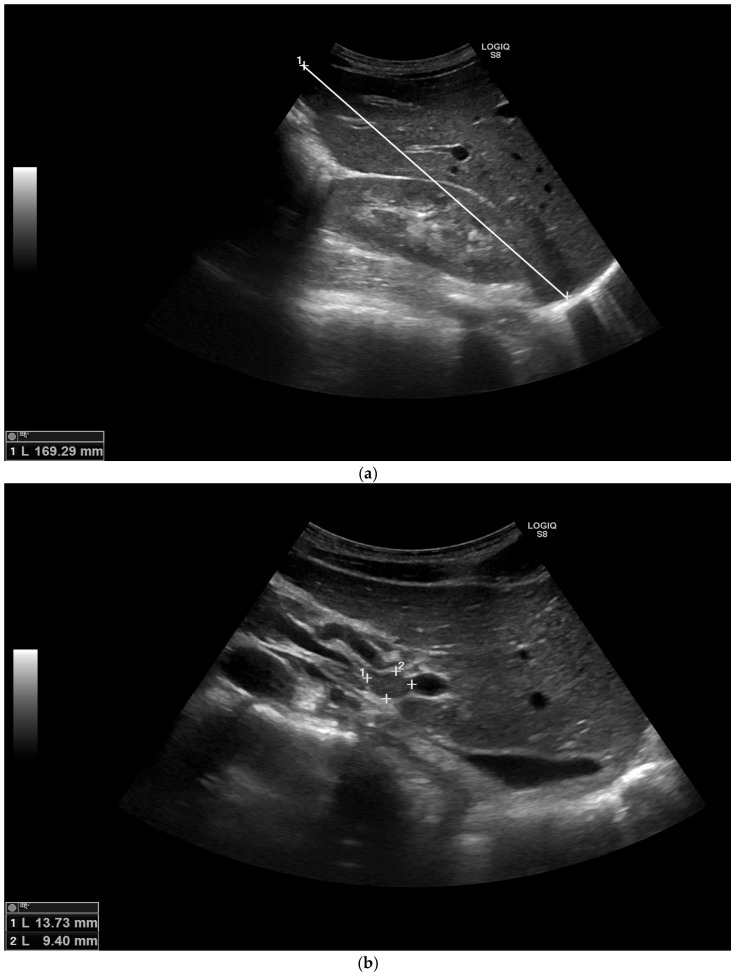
(a) Ultrasound of the abdomen showing hepatomegaly: liver diameter is 169.29 mm in the midclavicular line (normal range < 150.00 mm). (b) Ultrasound of the abdomen showing portal hepatic lymphadenopathy: lymph node dimensions 13.73 × 9.40 mm.
